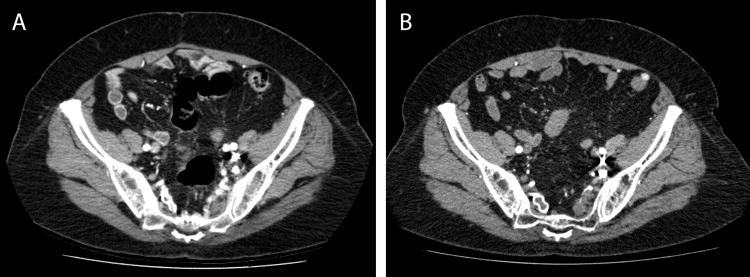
Contrast-enhanced CT of the pelvis, before (A) and 10 months after SBRT (B). On the post-radiotherapy study (B), note the persistence of bright embolization material along the left pelvic sidewall anterior to the left sacrum, but the resolution of enhancing AVM nidus and surrounding vessels.
